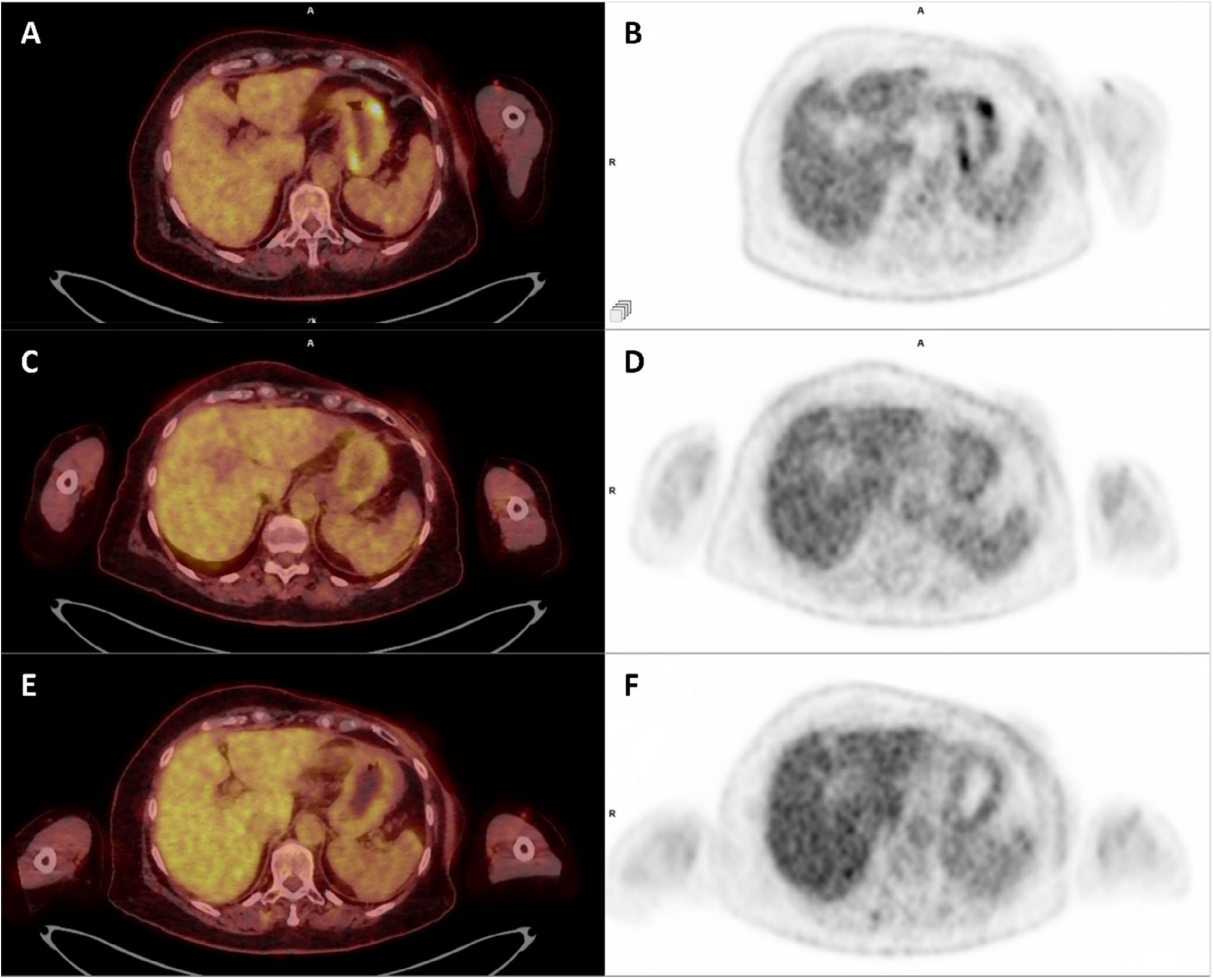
Comparison of FDG PET/CT images taken in July 2024 (A, B), October 2024 (C, D) and January 2025 (E, F), on axial fused PET/CT slice (A, C, E) and axial PET slice (B, D, F).
